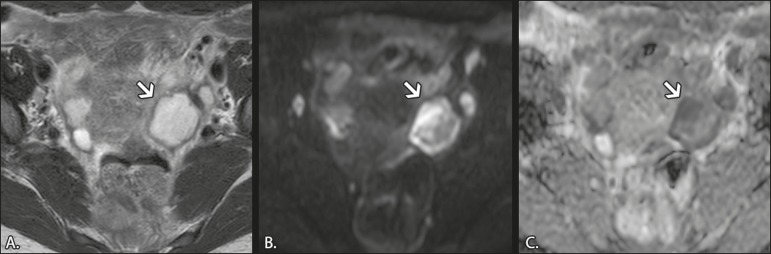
MRI of a 39-year-old female patient with a left tubo-ovarian
